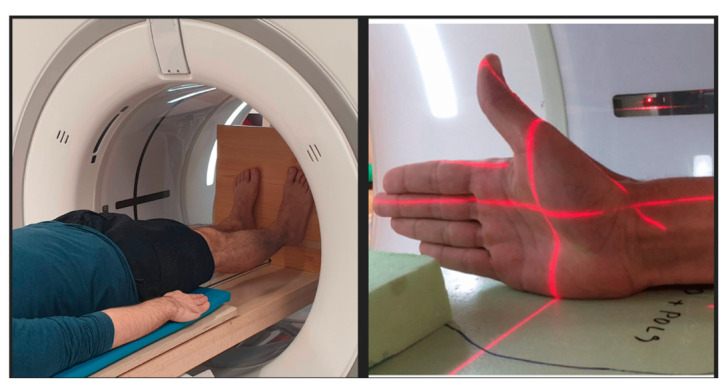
The figure shows the positioning in the gantry of the CT.
